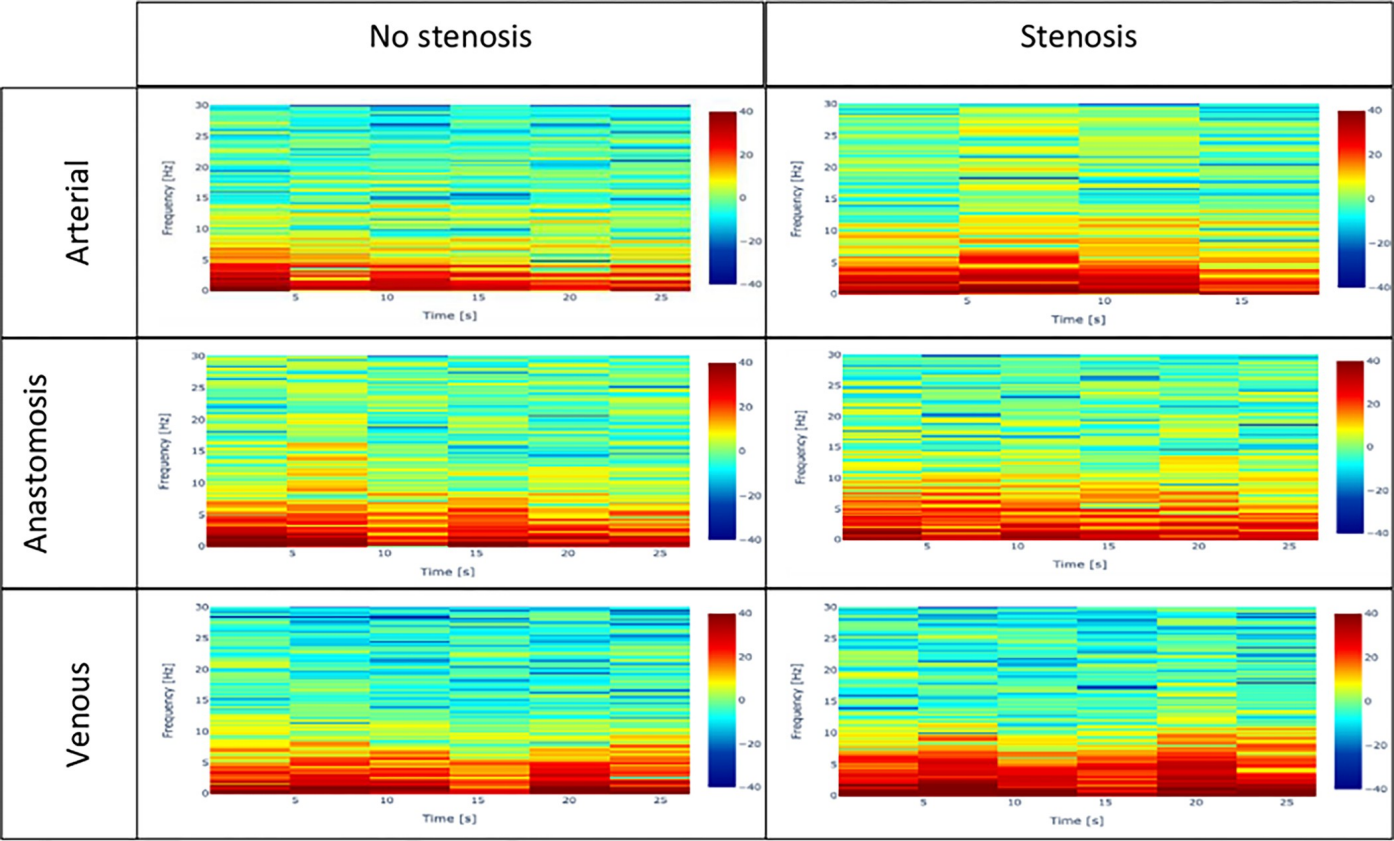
Spectrograms of arteriovenous flow (whole image) with and without stenosis. The spectrograms show the signals picked up in the whole image of arterial inflow, anastomosis, and venous outflow of fistulae with stenosis and no stenosis, which includes signals from the blood and the surrounding tissues. The Doppler readings in the vein with no stenosis are PSV (176 cm/s)–VF (475 ml/min)–diameter (2–5.8 mm) and with stenosis is diameter (<1 mm). In the anastomosis with no stenosis are PSV (386 cm/s)–diameter (4.4 mm) and with stenosis are PSV (500 cm/s)–diameter (2.4 mm). In the artery with no stenosis are PSV (187 cm/s)–VF (4950 ml/min)–diameter (5.8 mm) and with stenosis are PSV (315 cm/s)–VF (1800 ml/min)–diameter (5.2 mm).
